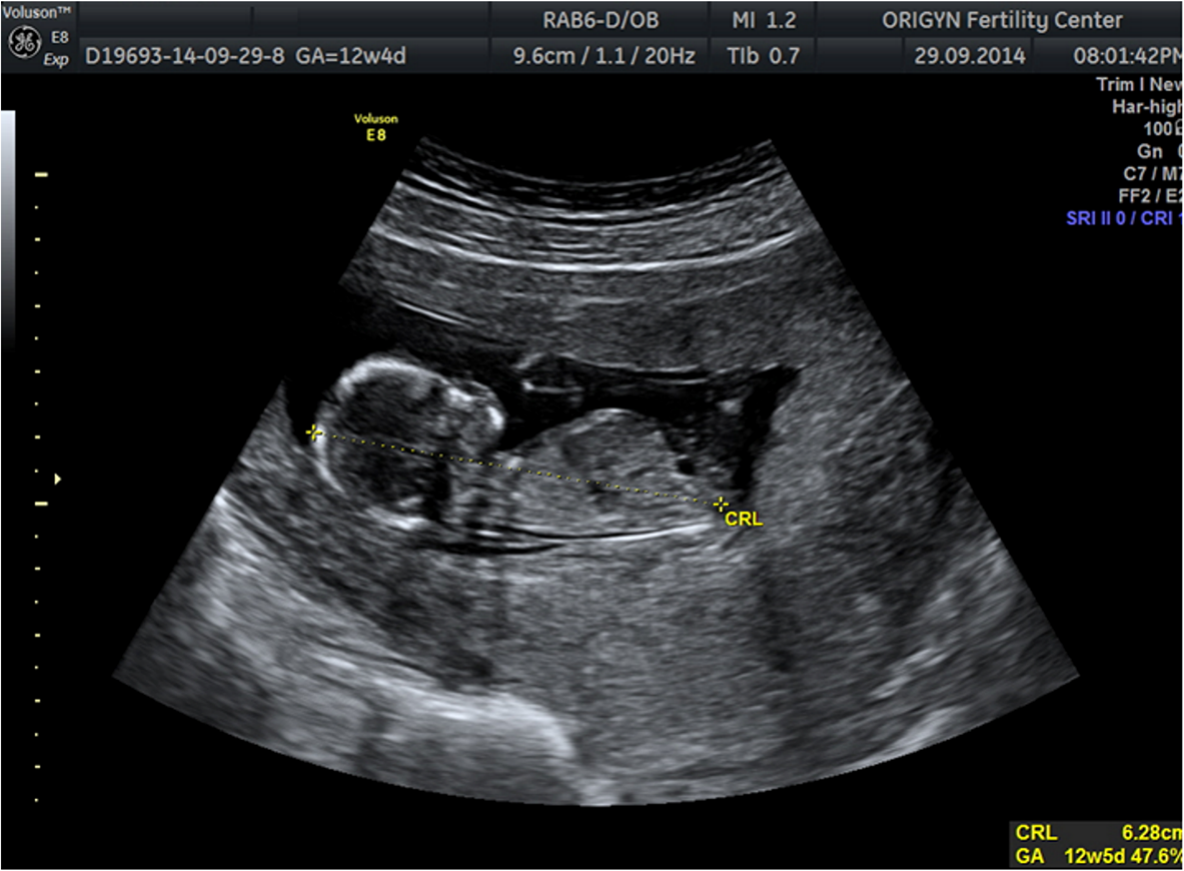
Ultrasound image of the fetus at 12 weeks and 2 days of gestation.
